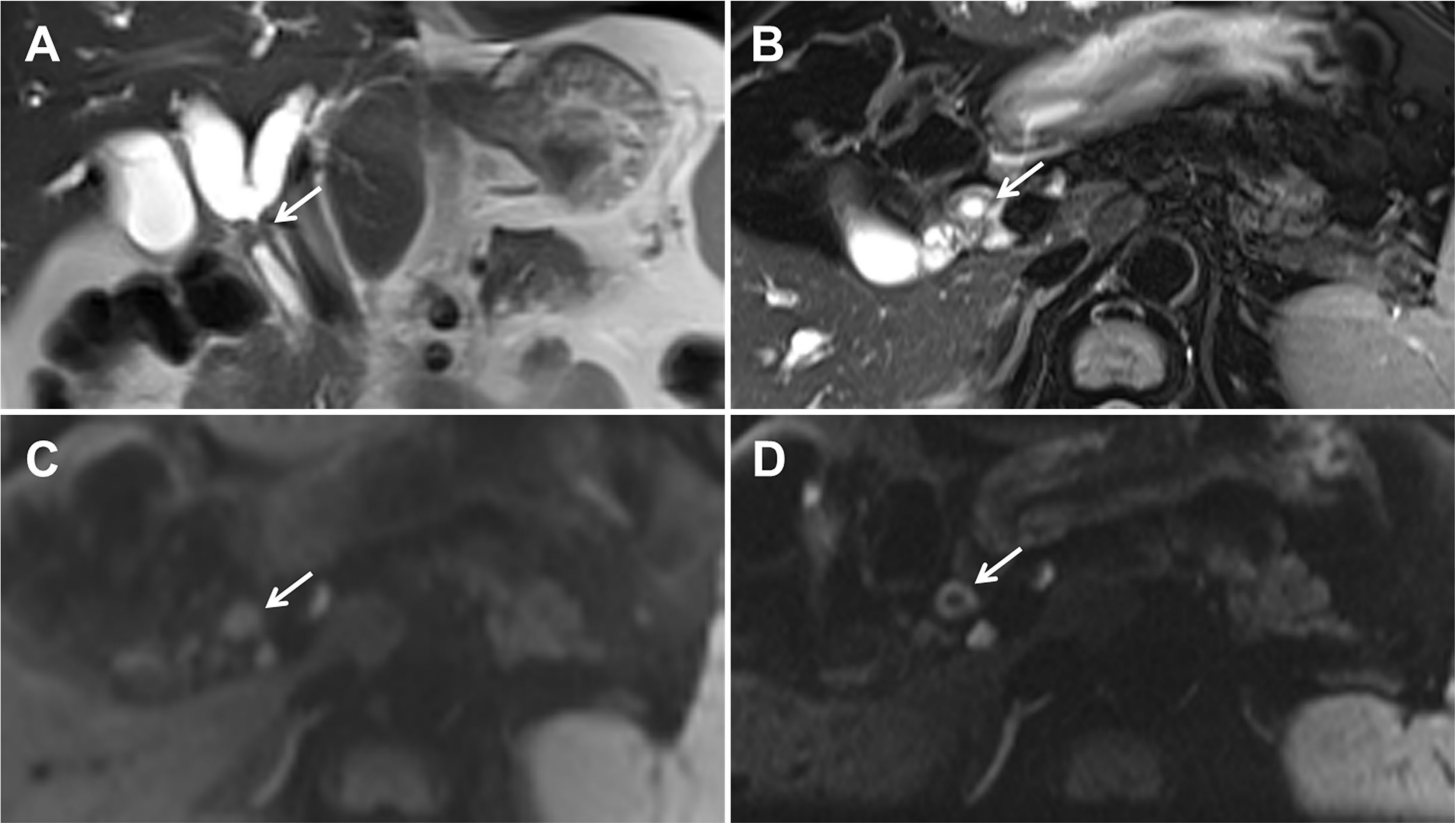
A 52-year-old man with Bismuth-Corlette type I hilar cholangiocarcinoma. Coronal (A) and axial (B) T2-weighted magnetic resonance imaging images demonstrating the hepatic duct with wall thickening and mild hyperintensities. Conventional echo-planar (c-EPI) diffusion-weighted imaging (DWI) (C) and zoomed echo-planar imaging (z-EPI) DWI (D) was obtained at b=800 s/mm^2^. The common hepatic duct lesion showed hyperintensities. In addition, the thickened common hepatic duct wall is better delineated on the z-EPI DWI image (arrow in D) compared with the c-EPI DWI image (arrow in C).
